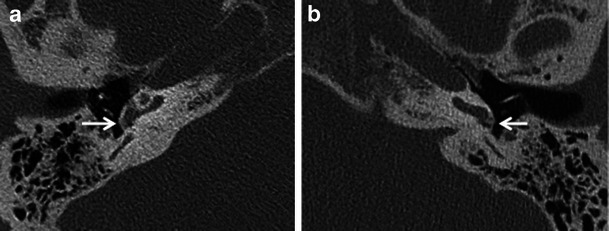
Axial HRCT images of the *right* (a) and *left* (b) temporal bone in a patient with bilateral fenestral otosclerosis. Otosclerotic plaques are noted causing bilateral round window narrowing (*arrows*), *right* more than *left*
